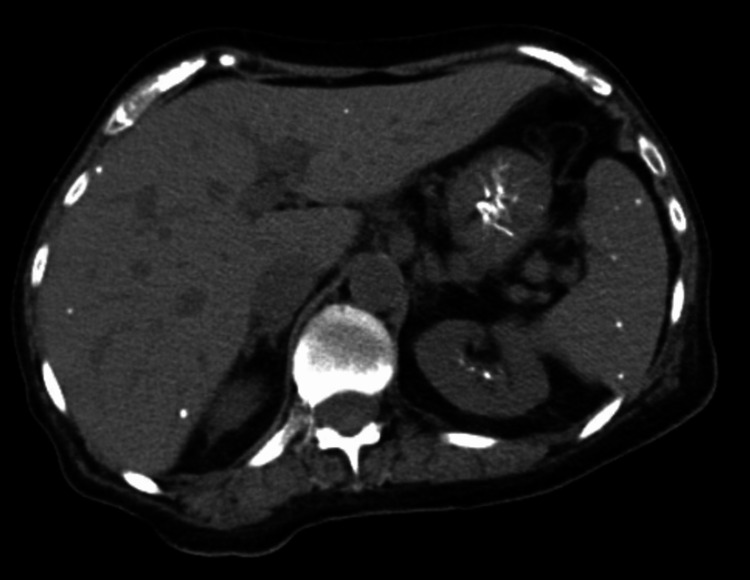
CT of the abdomen showing hepatic and splenic calcified granulomas, as well as nephrolithiasis
